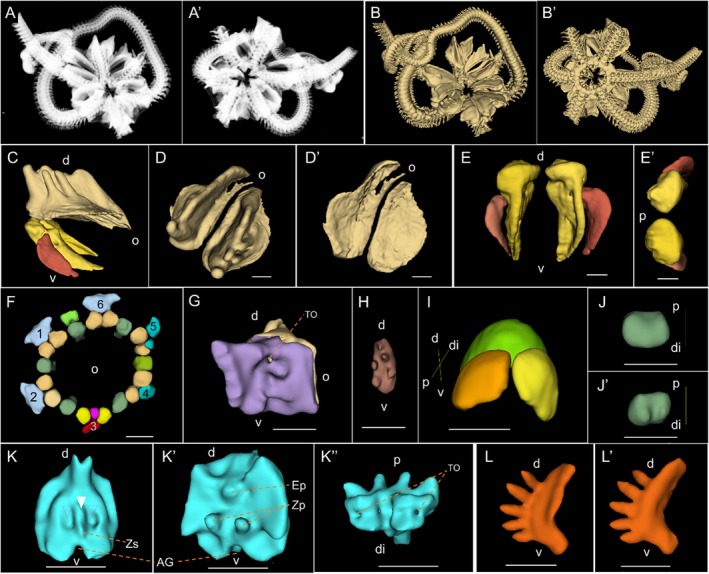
Micro CT segmentation of skeleton. (A, A') dorsal and ventral view of 3D rendering of the whole body; (B, B′) dorsal and ventral view of surface rendering of the whole body; (C–L') surface renderings of body parts. (C) combined view of radial shields (gold), adradial genital plates (yellow), and abradial genital plates (indian red); (D, D′) dorsal and ventral view of radial shield pair; (E, E') ventral view and radial view of adradial genital plates (yellow) and abradial genital plates (indian red); (F) dorsal view of oral shields (blue), adoral shields (yellow) and first ventral arm plate (green); (G) lateral view of two half jaws; (H) tooth plate with tooth or papilla; (I) dorsal view of dorsal arm plate (green) and split dorsal arm plate (orange and yellow); (J) ventral and dorsal view of ventral arm plate; (K, K′, K″) distal, proximal and ventral view of vertebrae; Zs: Zygosphene; Zp: Zygopophysis; Ep: Epanapophysis; AG: Ambulacral groov; (L, L') proximal and distal view of lateral plate with five spines. Scale bar is 1 mm; d, dorsal; v, ventral; di, distal; p, proximal; o, oral(mouth); TO, tubefeet opening.
